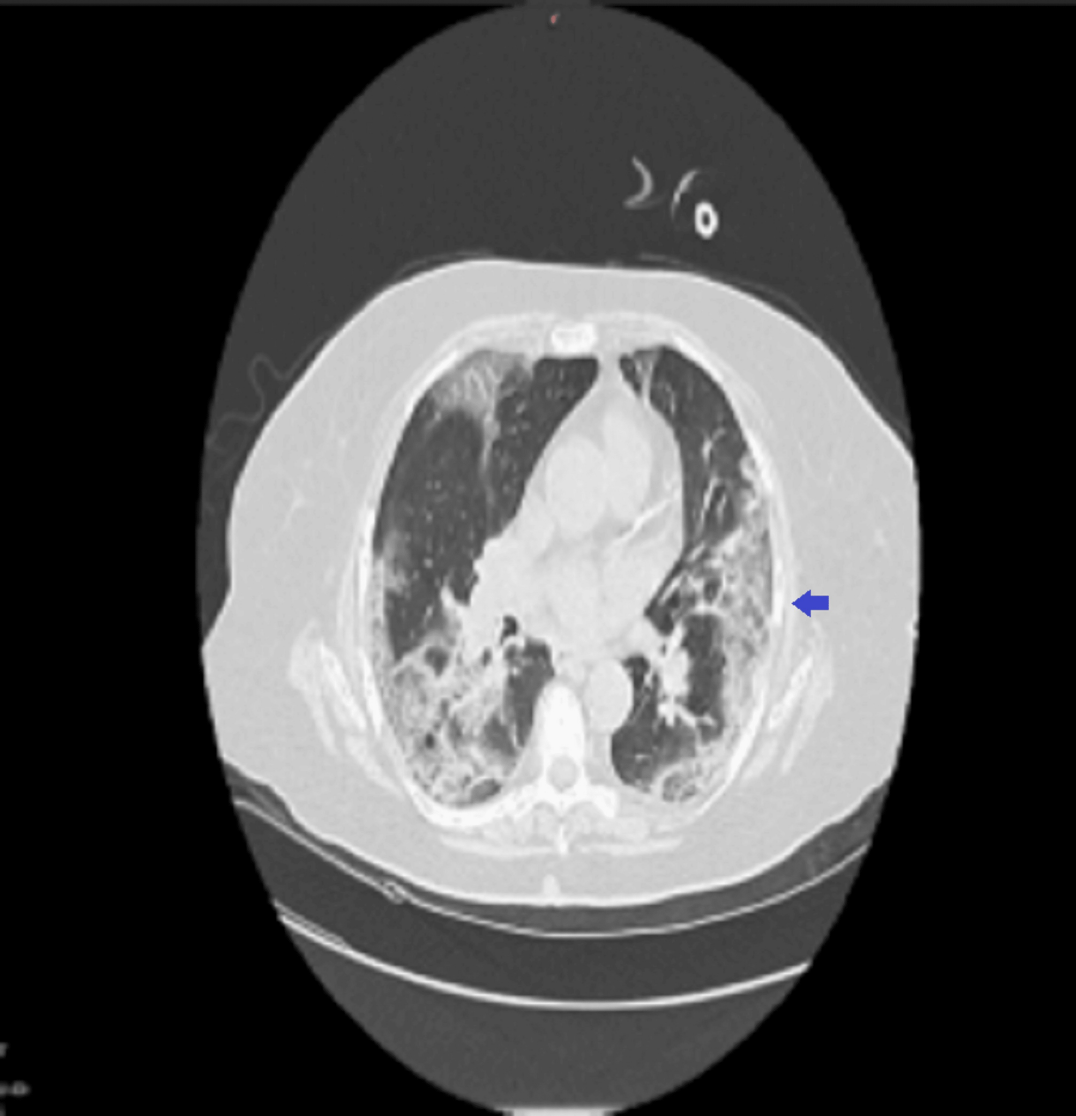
Chest CT showed extensive consolidations and ground-glass opacities in all lung lobes
